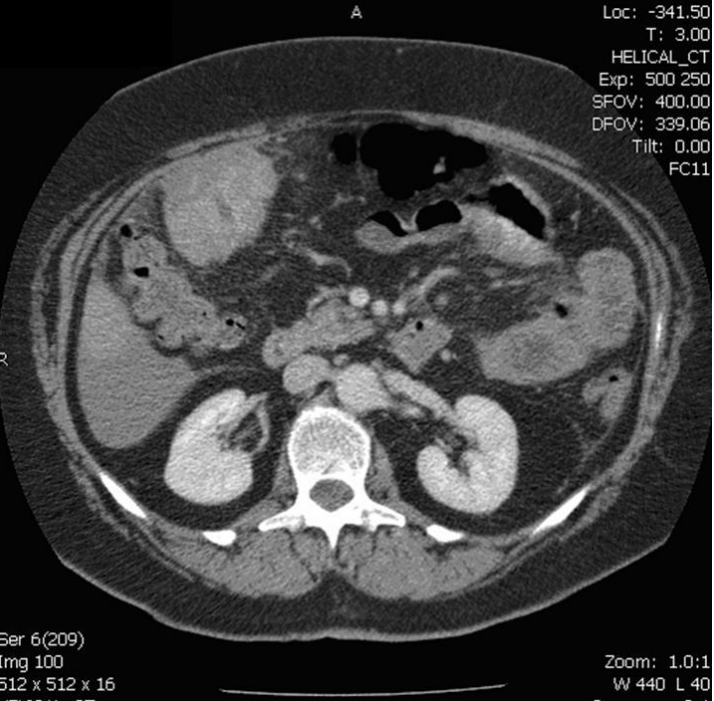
CT scan picture of enhancing lower extent of GIST visualized in relation to hepatic flexure of colon.
